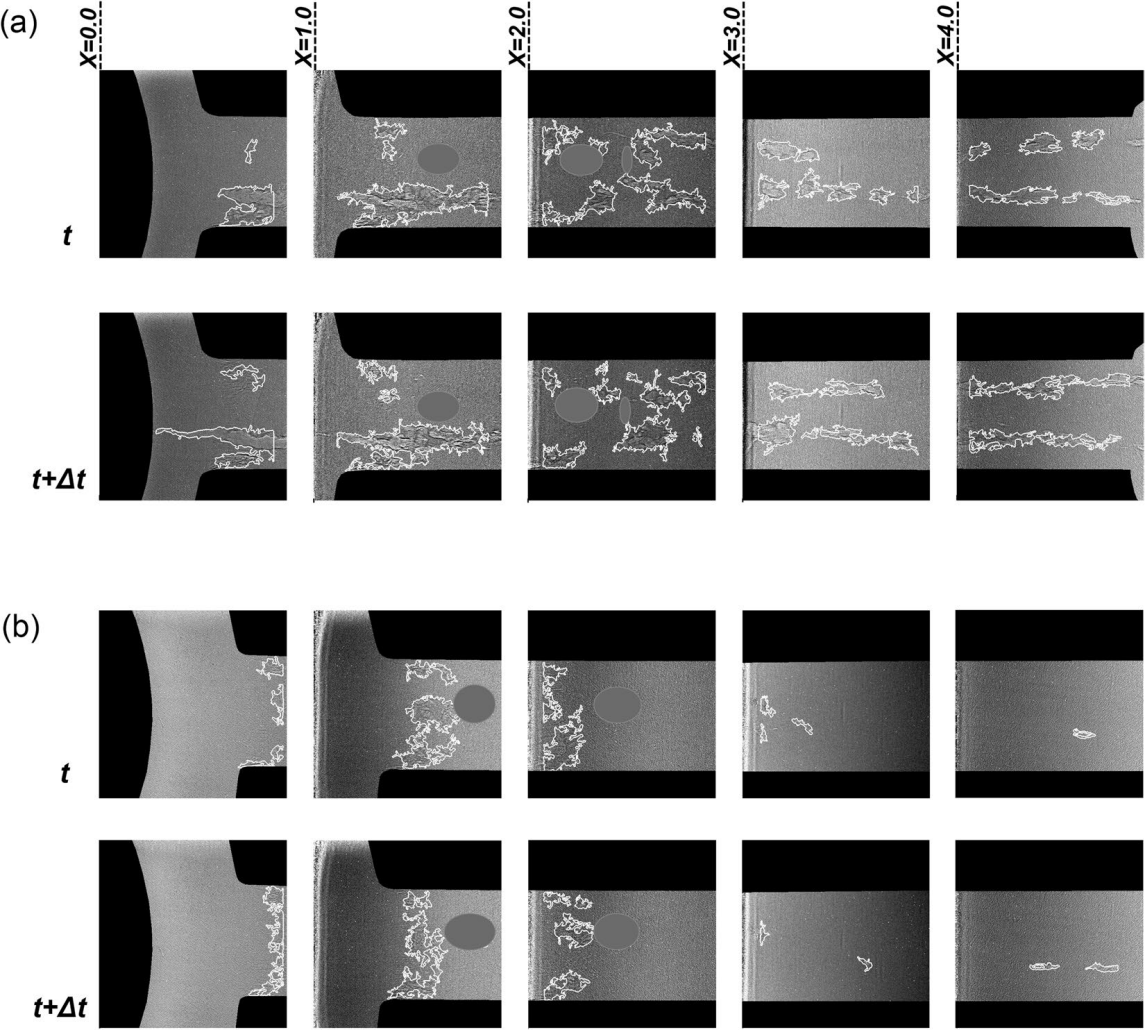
Top-view sequence of post-processed radiographs along the orifice length. The white line corresponds to the liquid-vapour interphase, while vertical white edges signify the extent of the irradiated region for which high signal-to-noise ratio could be obtained. Artefacts attributed to imperfections of the scintillator have been omitted in grey colour. (a) Needle lift equal to 0.5 mm for which string cavities arise in the orifice. (b) Needle lift equal to 1.0 mm giving rise to cloud cavitation. Both figures (a) and (b) present radiographs for CN = 3.0 and Re = 35500. The time interval *dt* is equal to 1.473 × 10^−5^ s.
